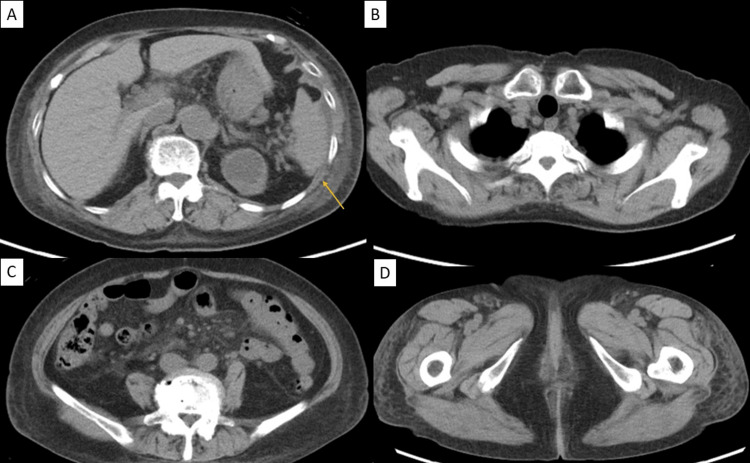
Computed tomography findings on admission. Whole-body computed tomography was performed to screen for underlying hematologic malignancies. (A) Abdominal CT showing a normal-sized spleen (arrow) without evidence of splenomegaly. (B) Chest, (C) abdominal, and (D) pelvic CT images demonstrating no significant lymphadenopathy in the cervical, axillary, mesenteric, or inguinal regions.
